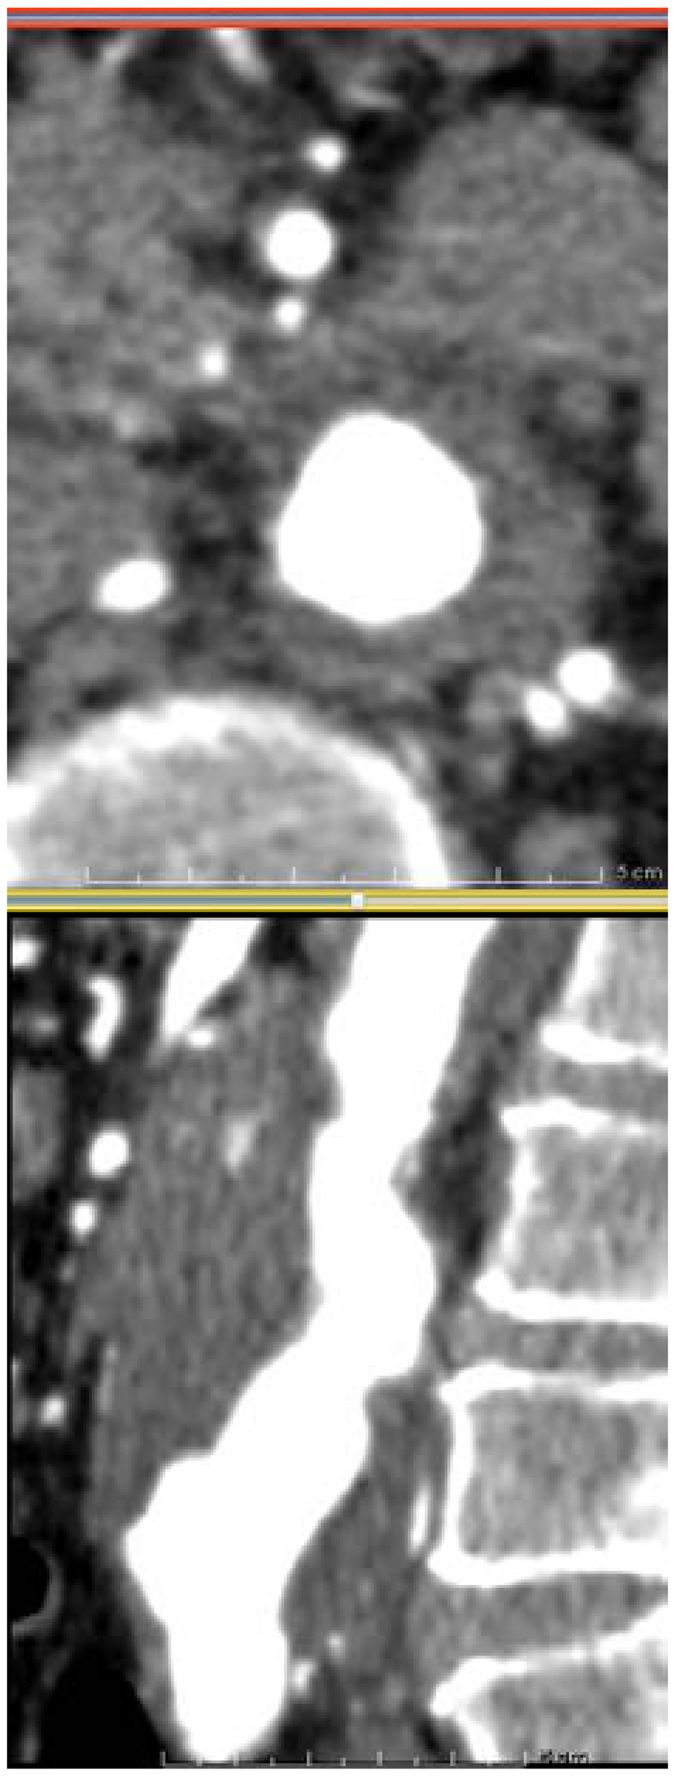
Case 2 used in reproducibility analysis. Axial (top) and sagittal (bottom) CT slices of the AAA. The poor contrast between the AAA and surrounding organs makes segmentation very challenging.
